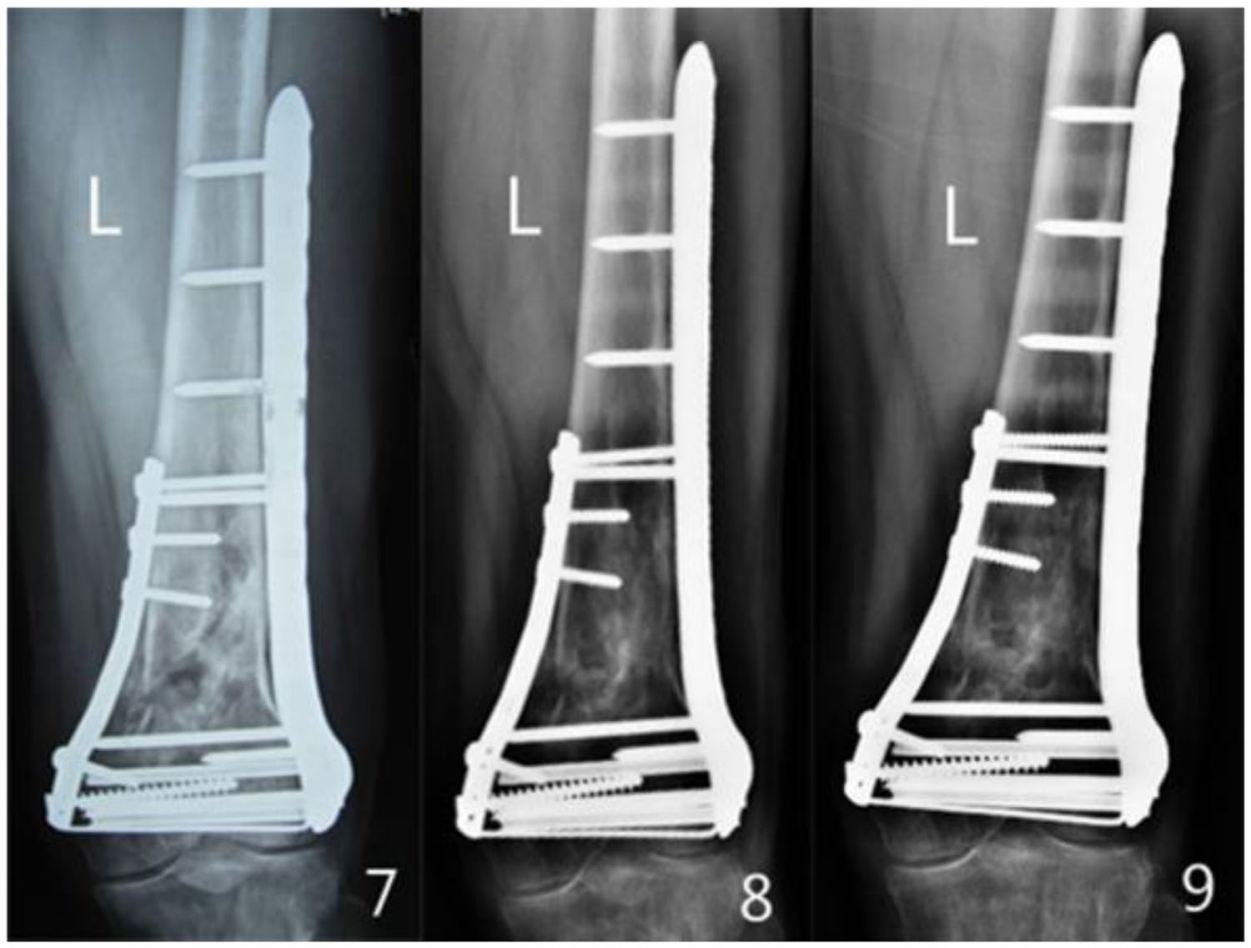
7, 8, 9 the X-ray of femur, taken by 1, 2, 3 years after operation.
